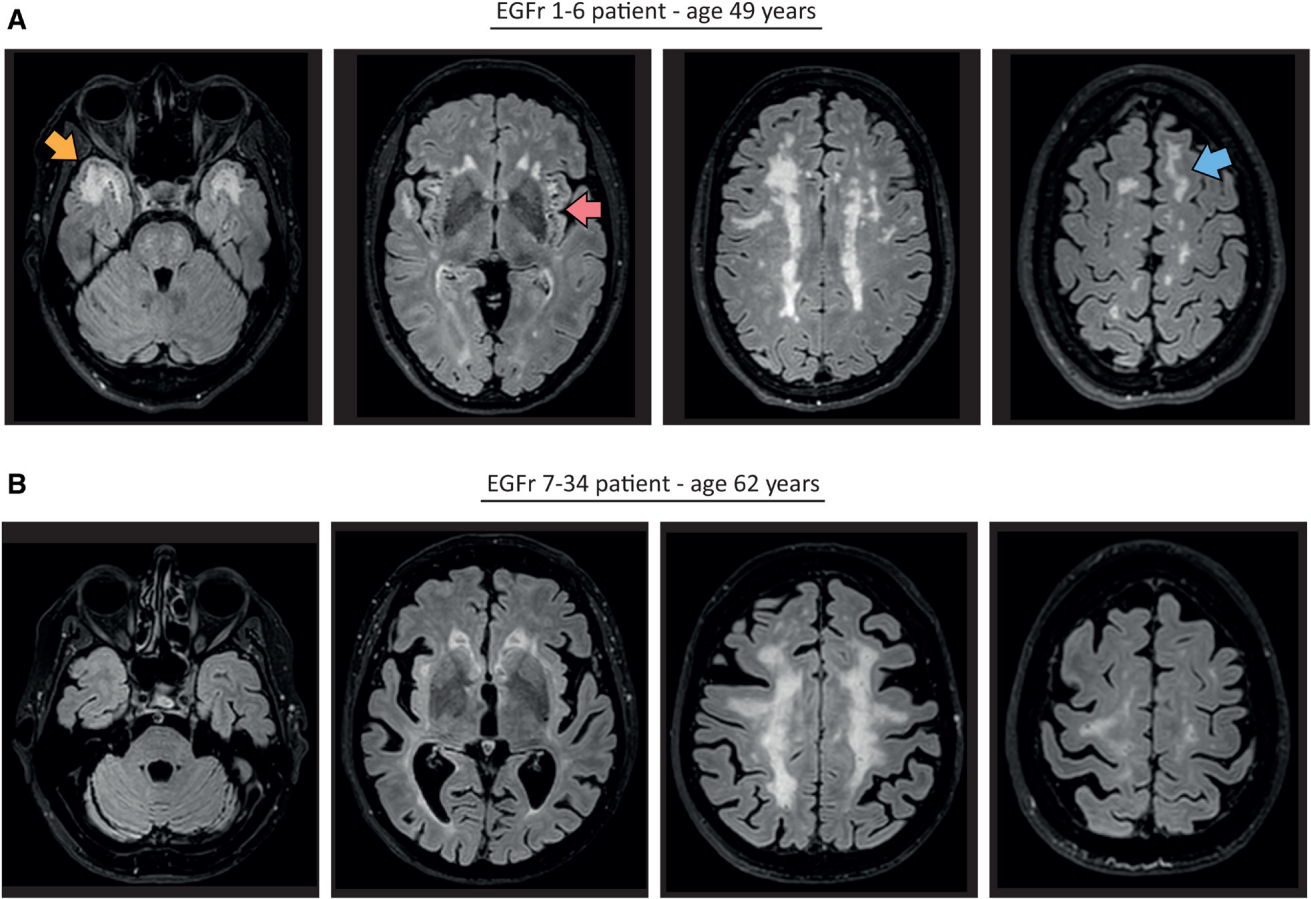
Representative brain magnetic resonance imaging (MRI) of an EGFr (epidermal growth factor-like repeat) 1–6 and an EGFr 7–34 patient with cerebral autosomal dominant arteriopathy with subcortical infarcts and leukoencephalopathy (CADASIL). A, Brain MRI FLAIR images of an EGFr 1–6 patient aged in his late 40s showing large confluent white matter hyperintensity (WMH) and a high burden of enlarged perivascular spaces (ePVS) in the anterior temporal lobes (yellow arrow), a high burden of ePVS in the subinsular regions (red arrow), and confluent WMH in the superior frontal gyri (blue arrow). B, Brain MRI fluid-attenuated inversion recovery images of an EGFr 7–34 patient aged in his early 60s with a similar total WMHv, but with no WMH in anterior temporal lobes, only small punctate foci of WMH in superior frontal gyri, and very low burden of ePVS in anterior temporal lobes and subinsular regions.
